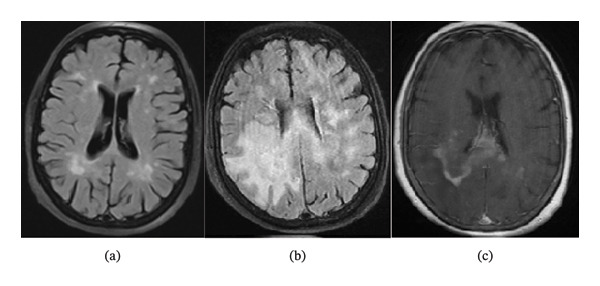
(a) (FLAIR): The patient’s previous typical MS lesions (2017); (b) (FLAIR) and (c) (T1 with contrast): Atypical lesions in recent brain MRI; diffuse large B‐cell lymphoma was confirmed.
